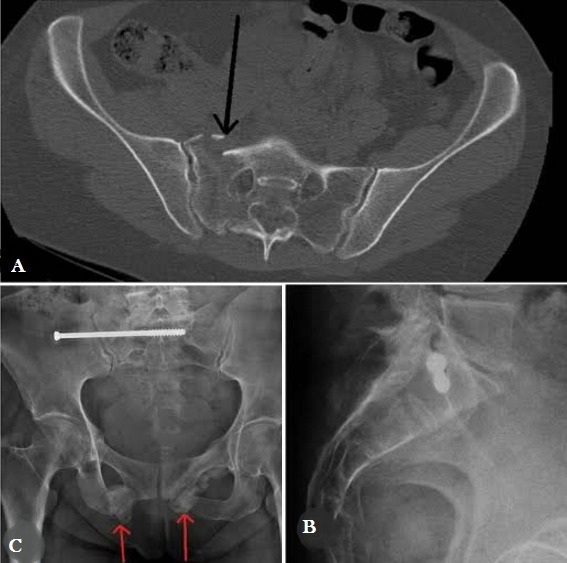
A) scanner du bassin montrant la fracture déplacée du sacrum; B) radiographie profil strict montrant le vissage sacro-iliaque; C) radiographie de face du bassin montrant la bonne consolidation du sacrum et des cadres obturateurs
